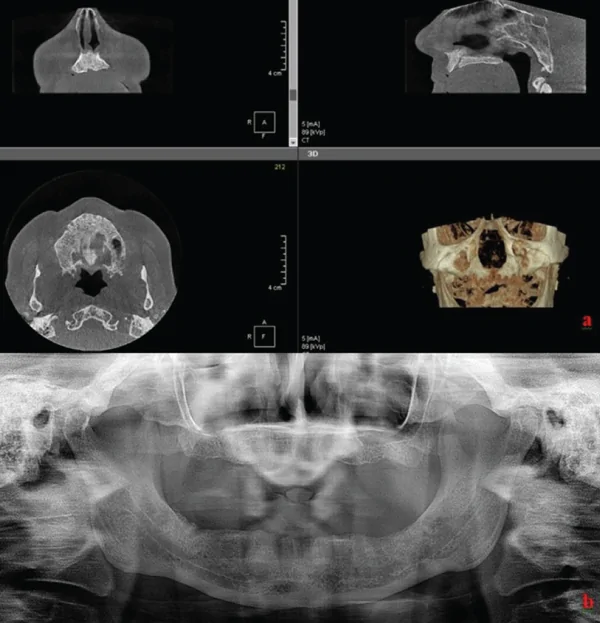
Radiographic examination of the lesion. (a) Cone‐beam computed tomography image demonstrating absence of osseous involvement. (b) Panoramic radiograph showing no radiographic evidence of osseous resorption in the anterior maxillary region.
